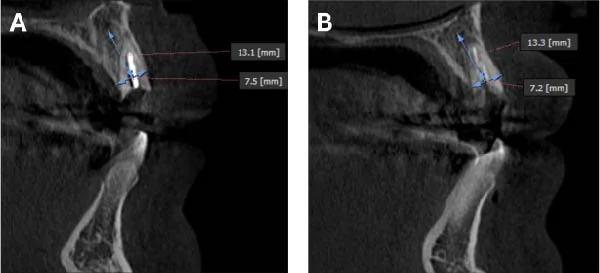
CBCT analysis of the alveolar ridge dimensions at the implant sites. (A) Upper right central incisor (#11) and (B) upper right lateral incisor (#12).
